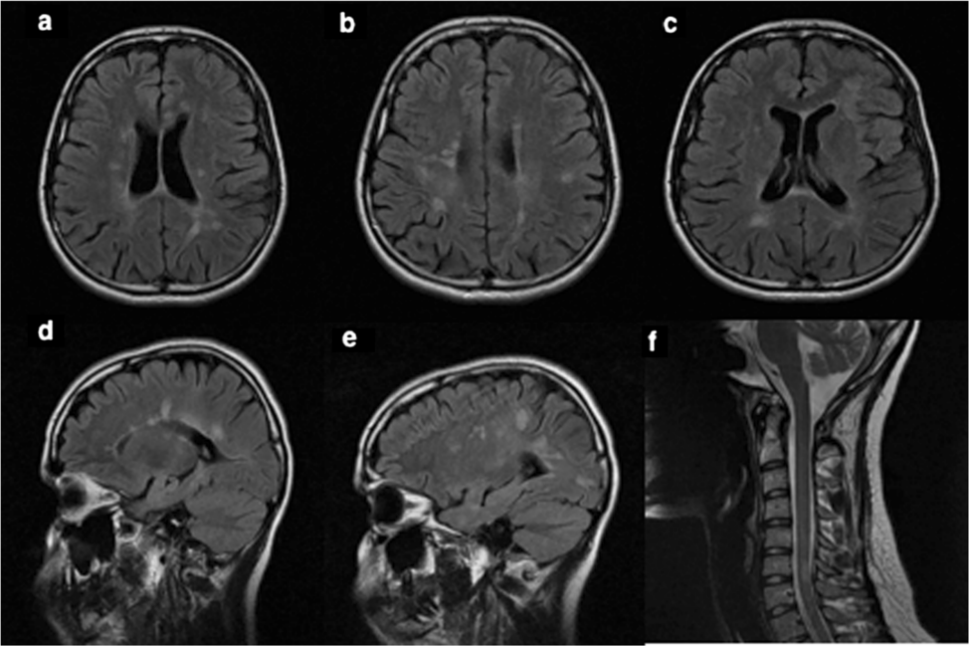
Follow-up MRI brain images (October 2012) showed an increase in the lesion load on axial (a-c) and Sagittal (d-e) T2/flair images when compared to the baseline MRI. Sagittal T2 spine images revealed a demyelinating plaque at C6 level (f).
